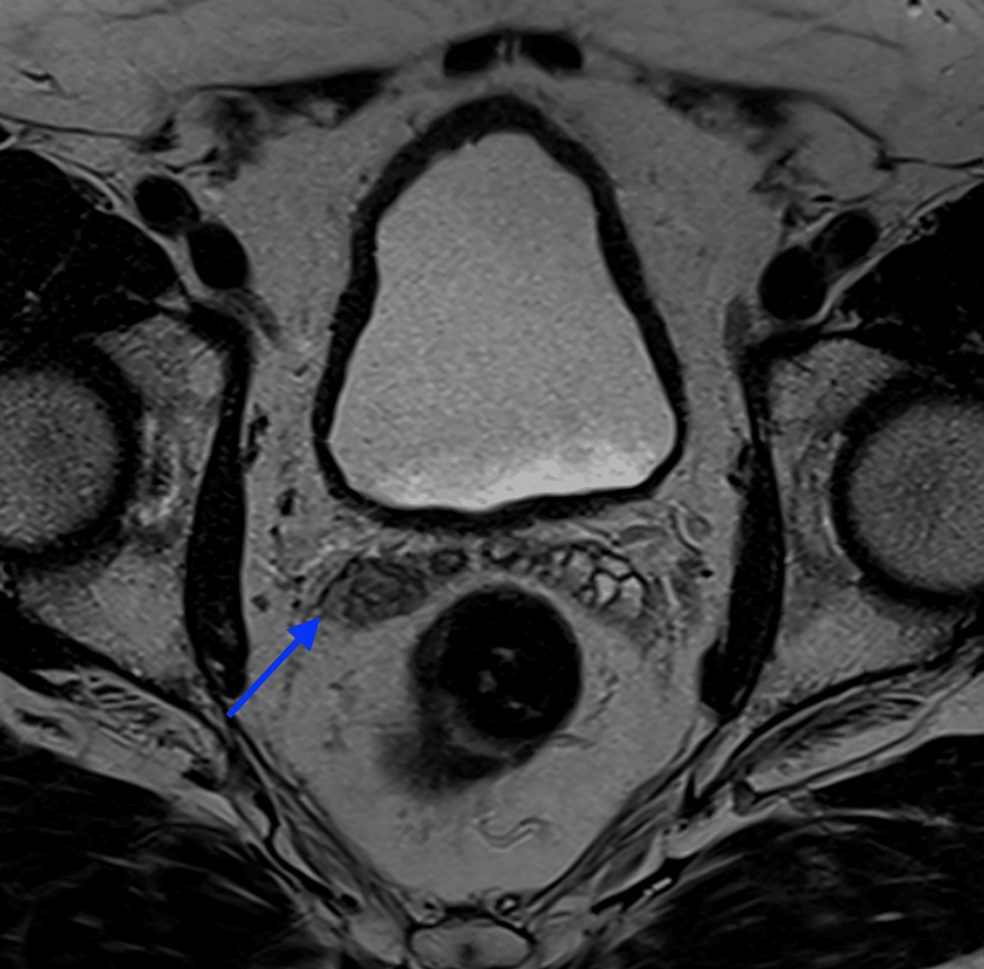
T2-weighted MRI showing low signal intensity in the right seminal vesicle (arrow)
